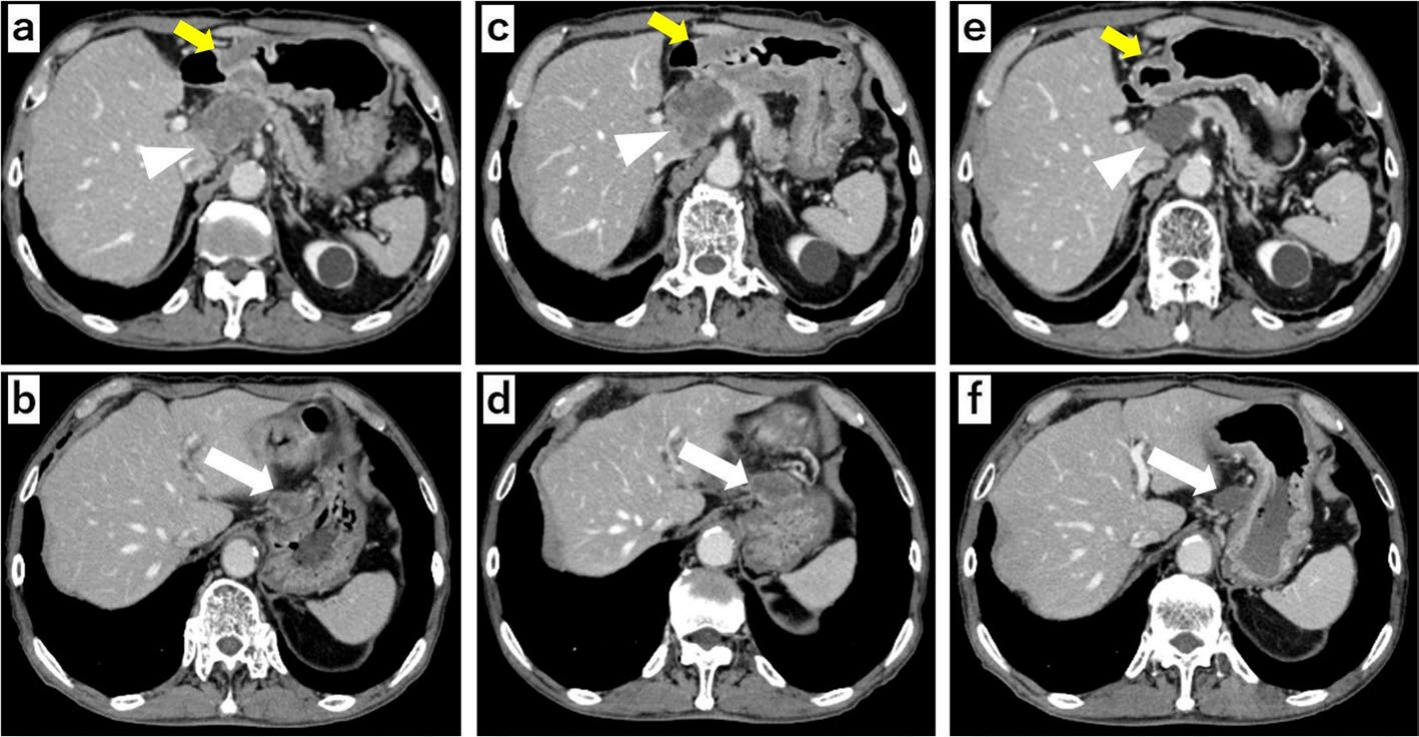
Contrast-enhanced CT scan. a, b Before chemotherapy (yellow arrows: primary tumor, arrowheads: anterior superior lymph nodes of the common hepatic artery, white arrows: lesser curvature lymph nodes). c, d After three courses of mFOLFOX6. e, f After three courses of pembrolizumab
